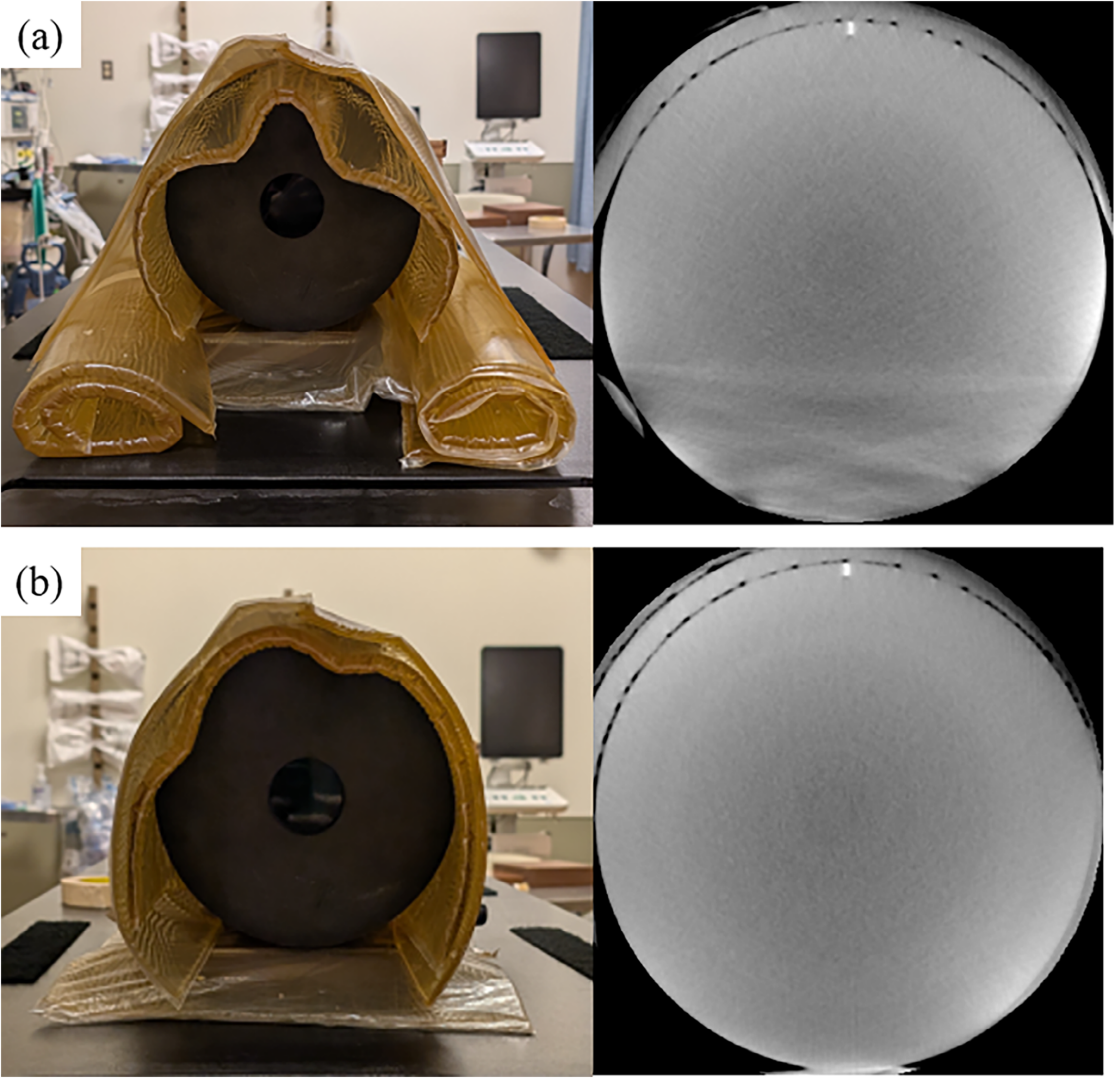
The experiment setup and the CT images in the uniformity module in the MDL protocol: (a) is a full bolus, (b) is a half bolus. (WL/WW [‐40, 500]).
